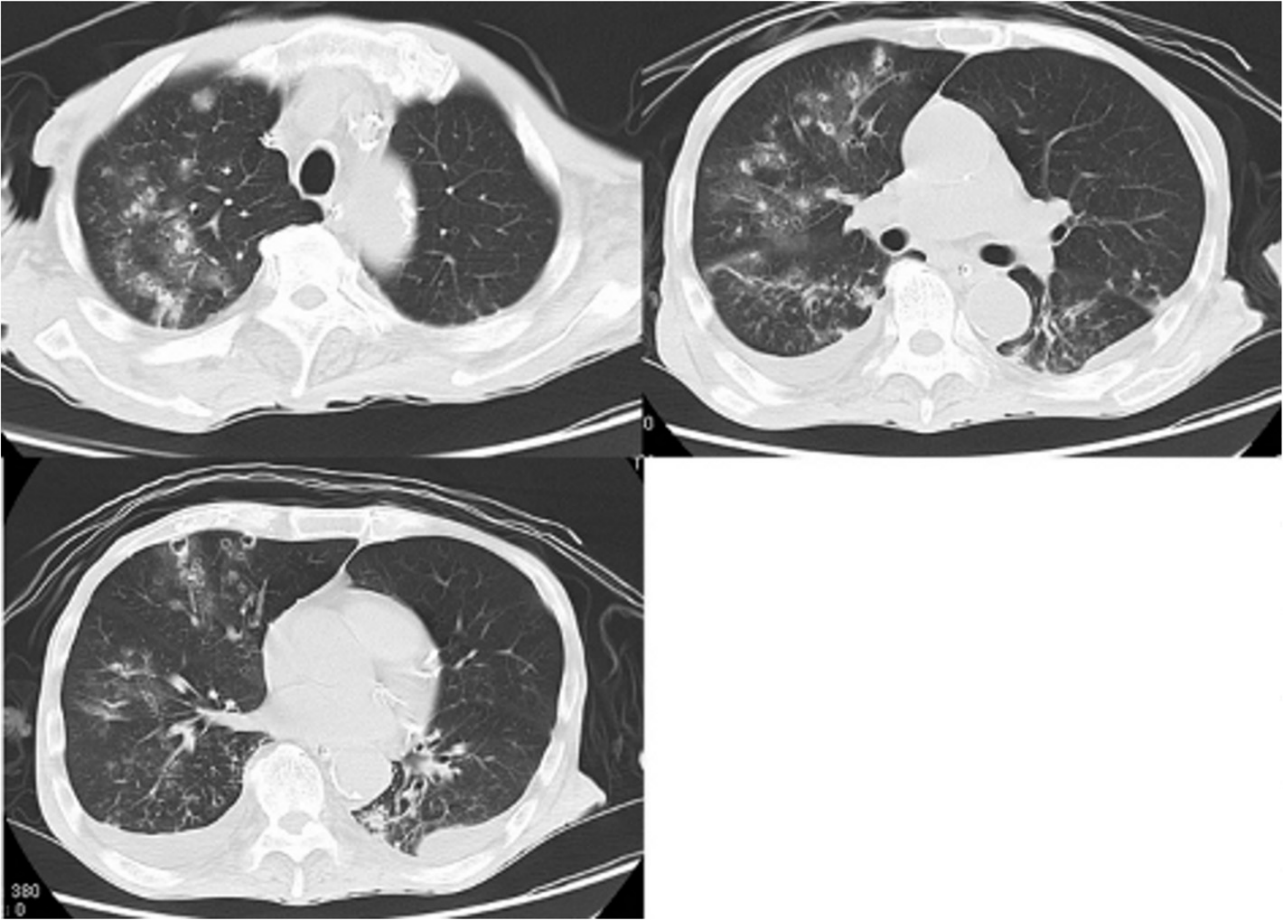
Multiple small cavitary lesions and nodules. Chest CT images obtained on the 14^th^ day of admission showed multiple small cavitary lesions and nodules surrounded by ground-glass opacity, and also bilateral pleural effusion. These lung abnormalities seemed to be in a peribronchovascular distribution.
